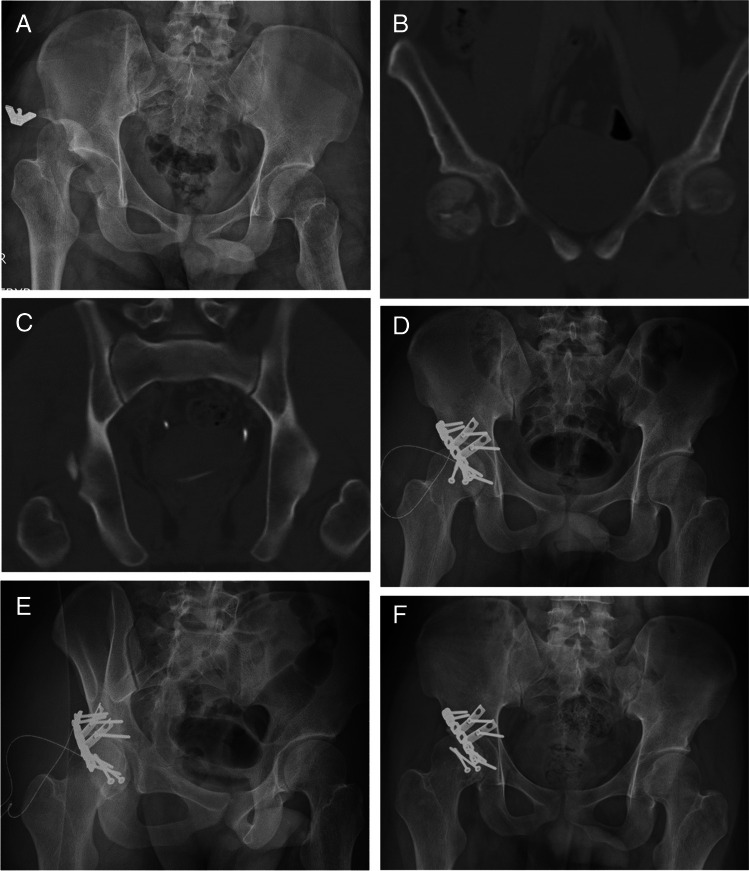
Representative case of an 18-year-old man involved in a motorbike accident. A Right hip traumatic fracture-dislocation of an 18-year-old male after a motorbike accident. B and C CT scan after dislocation reduction showing femoral head and acetabular posterior wall fracture. The patient was operated with the modified Hardinge approach. D Post-operative anteroposterior, and E obturator oblique radiographs demonstrating congruent hip joint. F Radiograph 14 months after the operation showing no signs of osteonecrosis or osteoarthritis. CT, computed tomography
